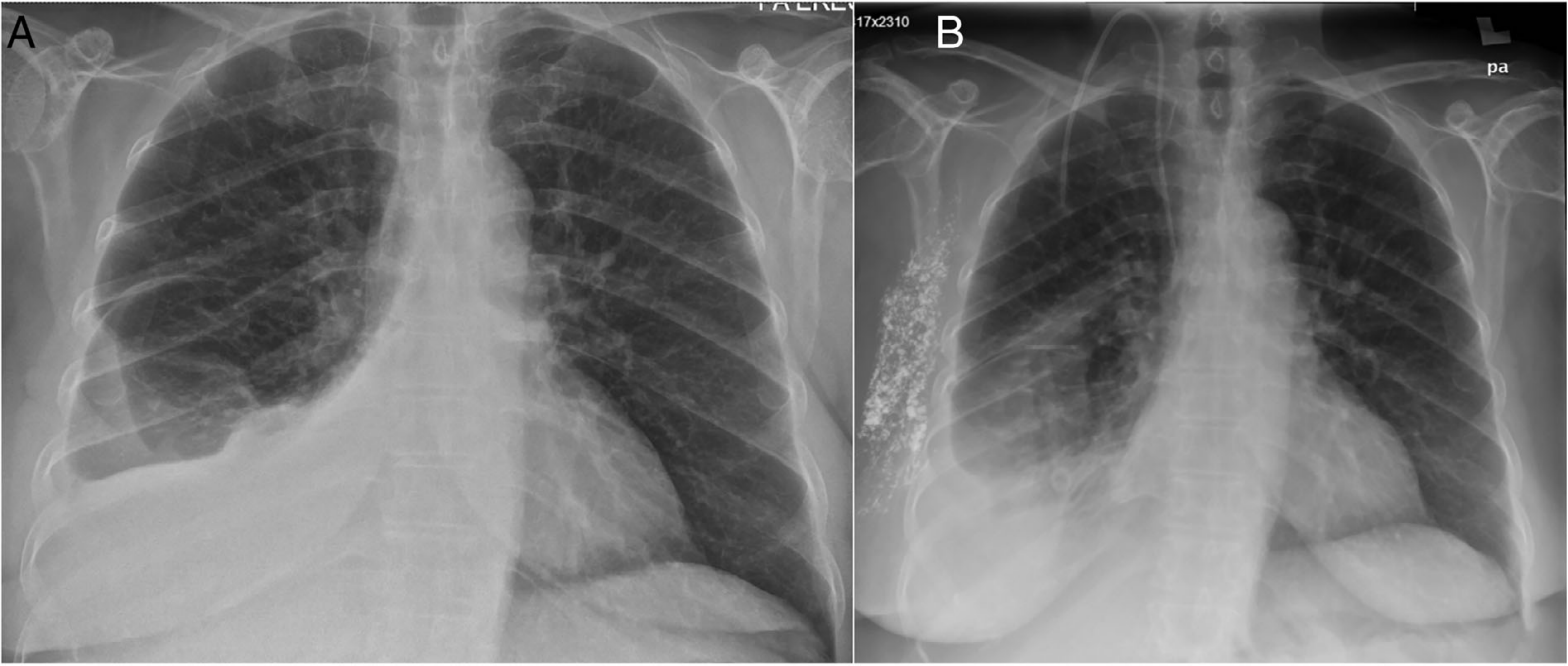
(A) Chest radiograph on admission showing moderate right pleural effusion. (B) Chest radiograph with resolution of right chylothorax. Also seen is the contrast medium from lymphangiogram and a central line in situ.
